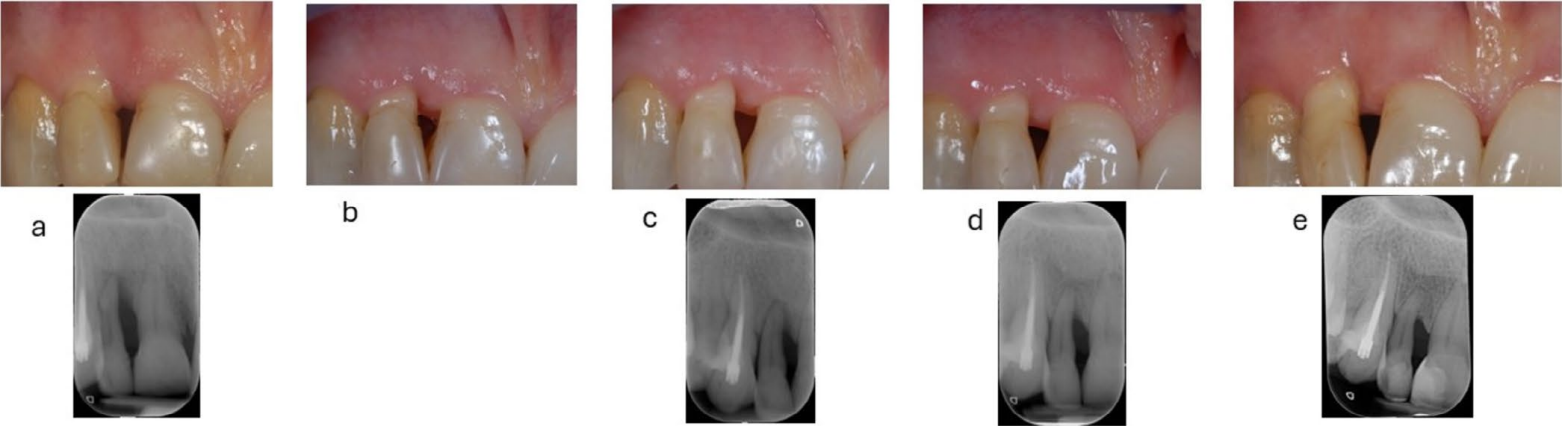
Sequence of images and radiographs showing the healing development following non‐surgical periodontal therapy (NSPT). (a) Baseline condition of a site presenting with inflammation, swelling, exudate and a deep intrabony defect associated with a 10‐mm pocket. This site is amenable to periodontal regeneration. (b) 6 weeks after NSPT at first re‐evaluation it is evident the resolution of gingival inflammation and the contraction of the marginal soft tissues. (c) Second re‐evaluation at 3 months showing further remodelling of the soft tissues. The radiograph shows an initial re‐mineralisation of the intrabony defect associated with 5‐mm pocket depth (PD). (d) Third re‐evaluation at 1 year showing a considerable re‐mineralisation associated with a PD of 4 mm. The clinical condition of the site after NSPT does not indicate the application of regenerative surgery. (e) 10 years follow up with stability of hard and soft tissues.
